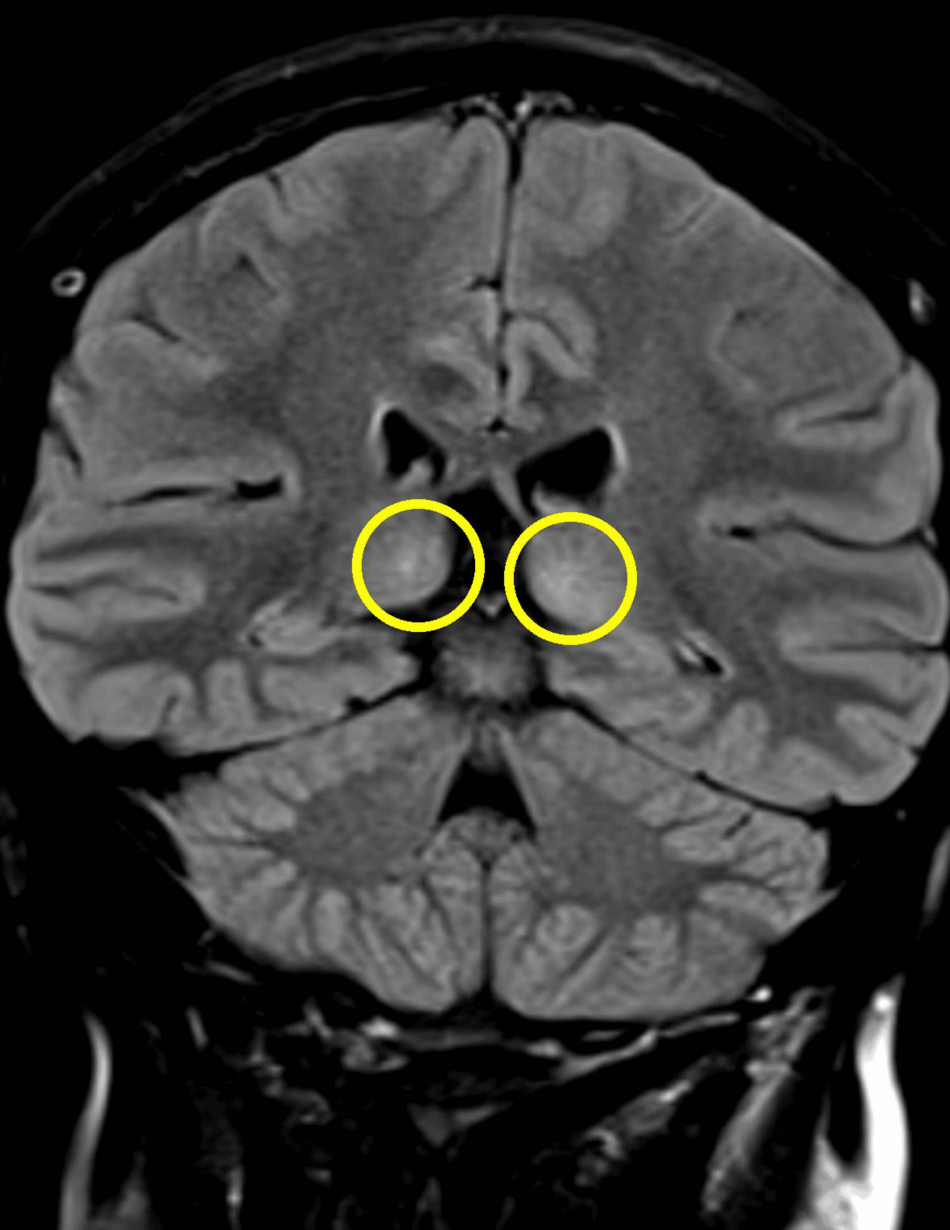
Coronal FLAIR MRI Brain Image Coronal FLAIR MRI sequence showing bilateral thalamic hyperintensity, with increased signal intensity involving the medial thalami. These changes support the diagnosis of atezolizumab-induced encephalitis. FLAIR: Fluid-Attenuated Inversion Recovery
